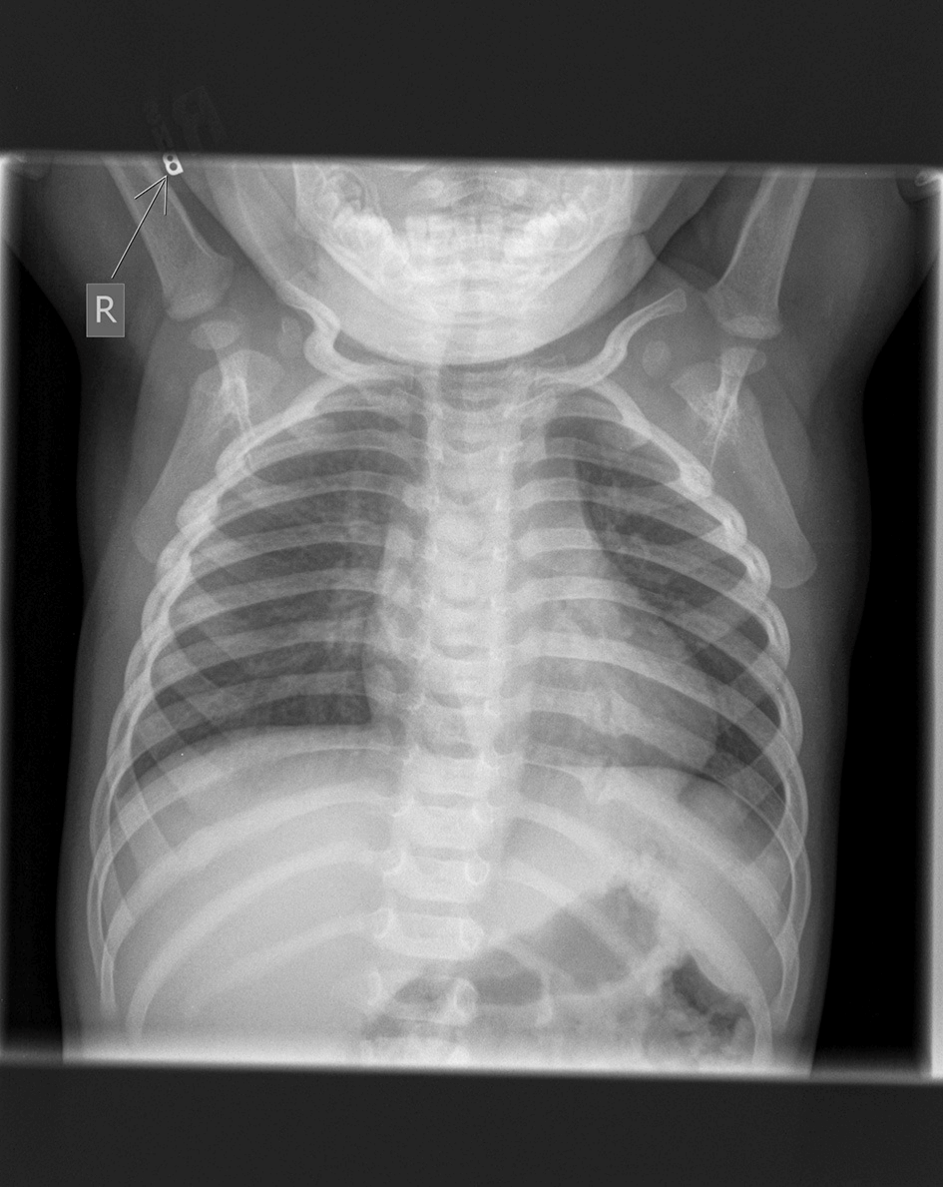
Chest x-ray showing multiple rib fractures from child abuse. Fractures of ribs 3, 4, and 10 on the right and ribs 8, 9, and 10 on the left. Image courtesy of Nancy Harper, MD Masonic Children's Hospital, Minneapolis, MN and Sonja Eddleman, RN, Driscoll Children's Hospital, Corpus Christi, TX.
